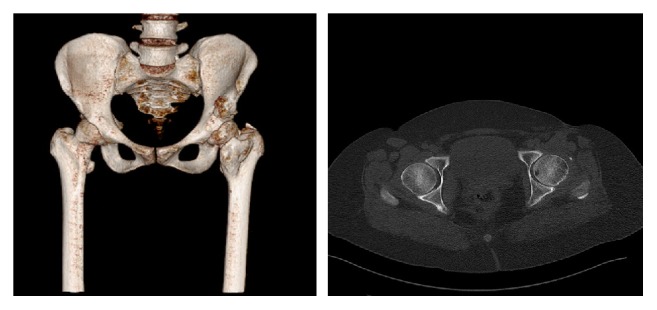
Post-op 2nd-year radiographic evaluation with 3D and axial view of CT.
